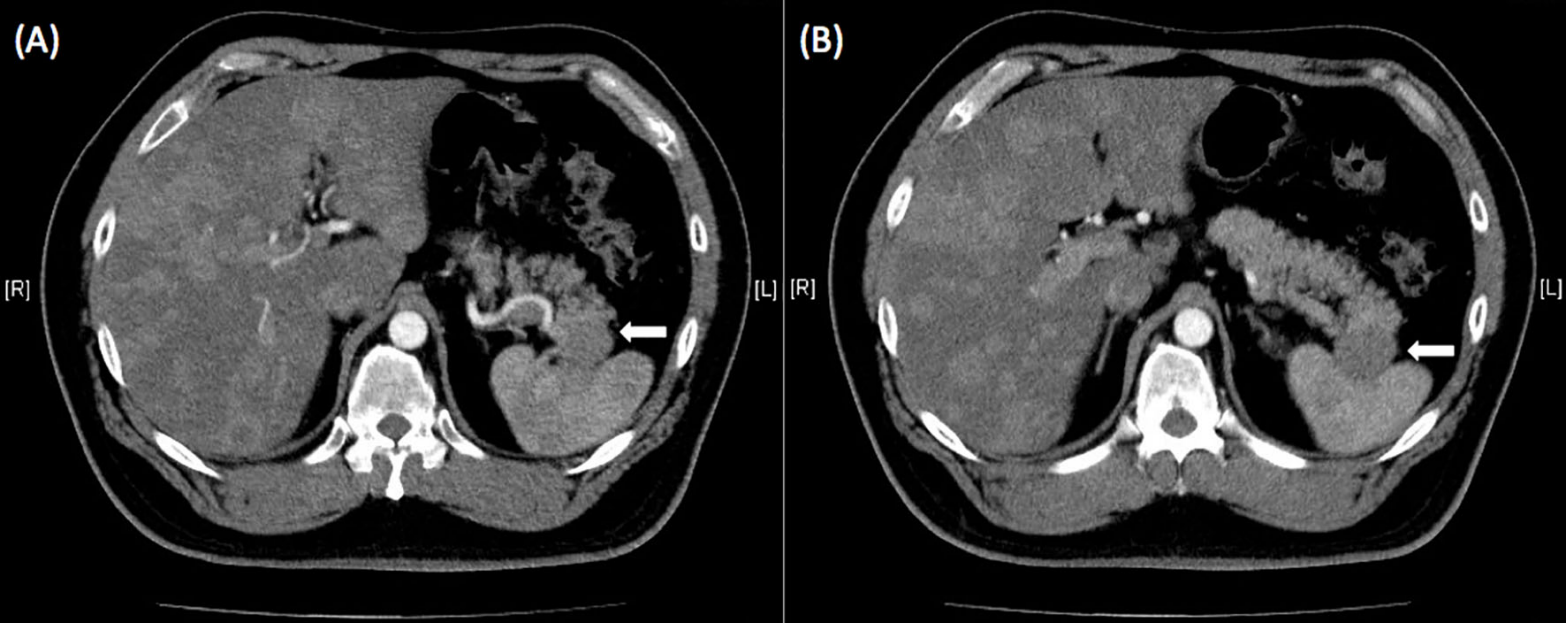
(A) Abdominal computed tomography showed a pancreatic body tumor approximately 1.5 cm in size associated with liver tumors in the right and left lobes. Adrenal glands were normal without enlargement. (B) A suspected tumor at the pancreatic tail was disclosed on enhanced abdominal computed tomography (white arrow).
